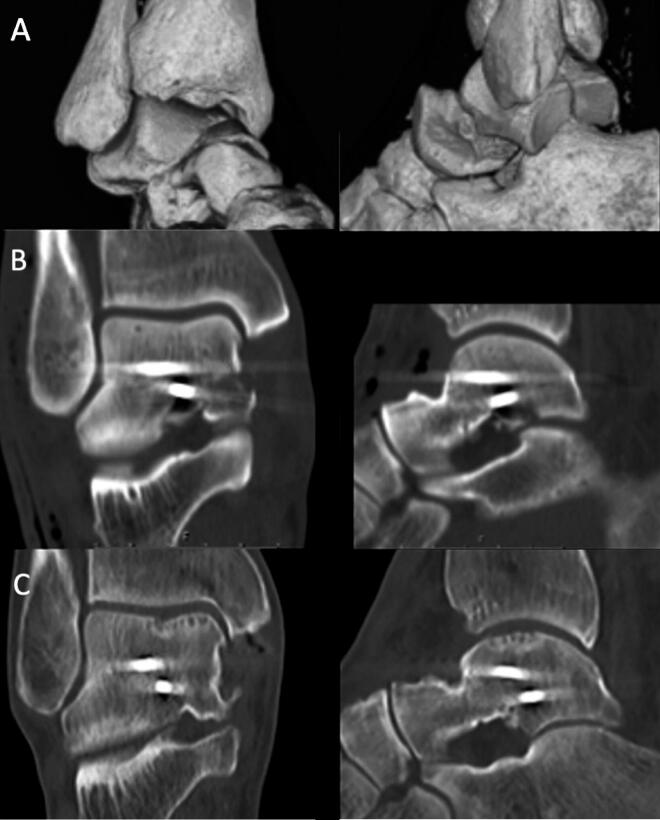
CT reconstruction demonstrating a Hawkins type 2b fracture with comminution of the talar dome (A) in a 60-year-old man after a low-energy fall. The fracture was reduced and fixed with 2 screws (B), followed by 6 weeks in a non-weight-bearing cast. No antiresorptive treatment was given. At 11.8 years postoperatively, the fracture was healed and the talar dome showed partial collapse (C). (SEFAS of 24).
